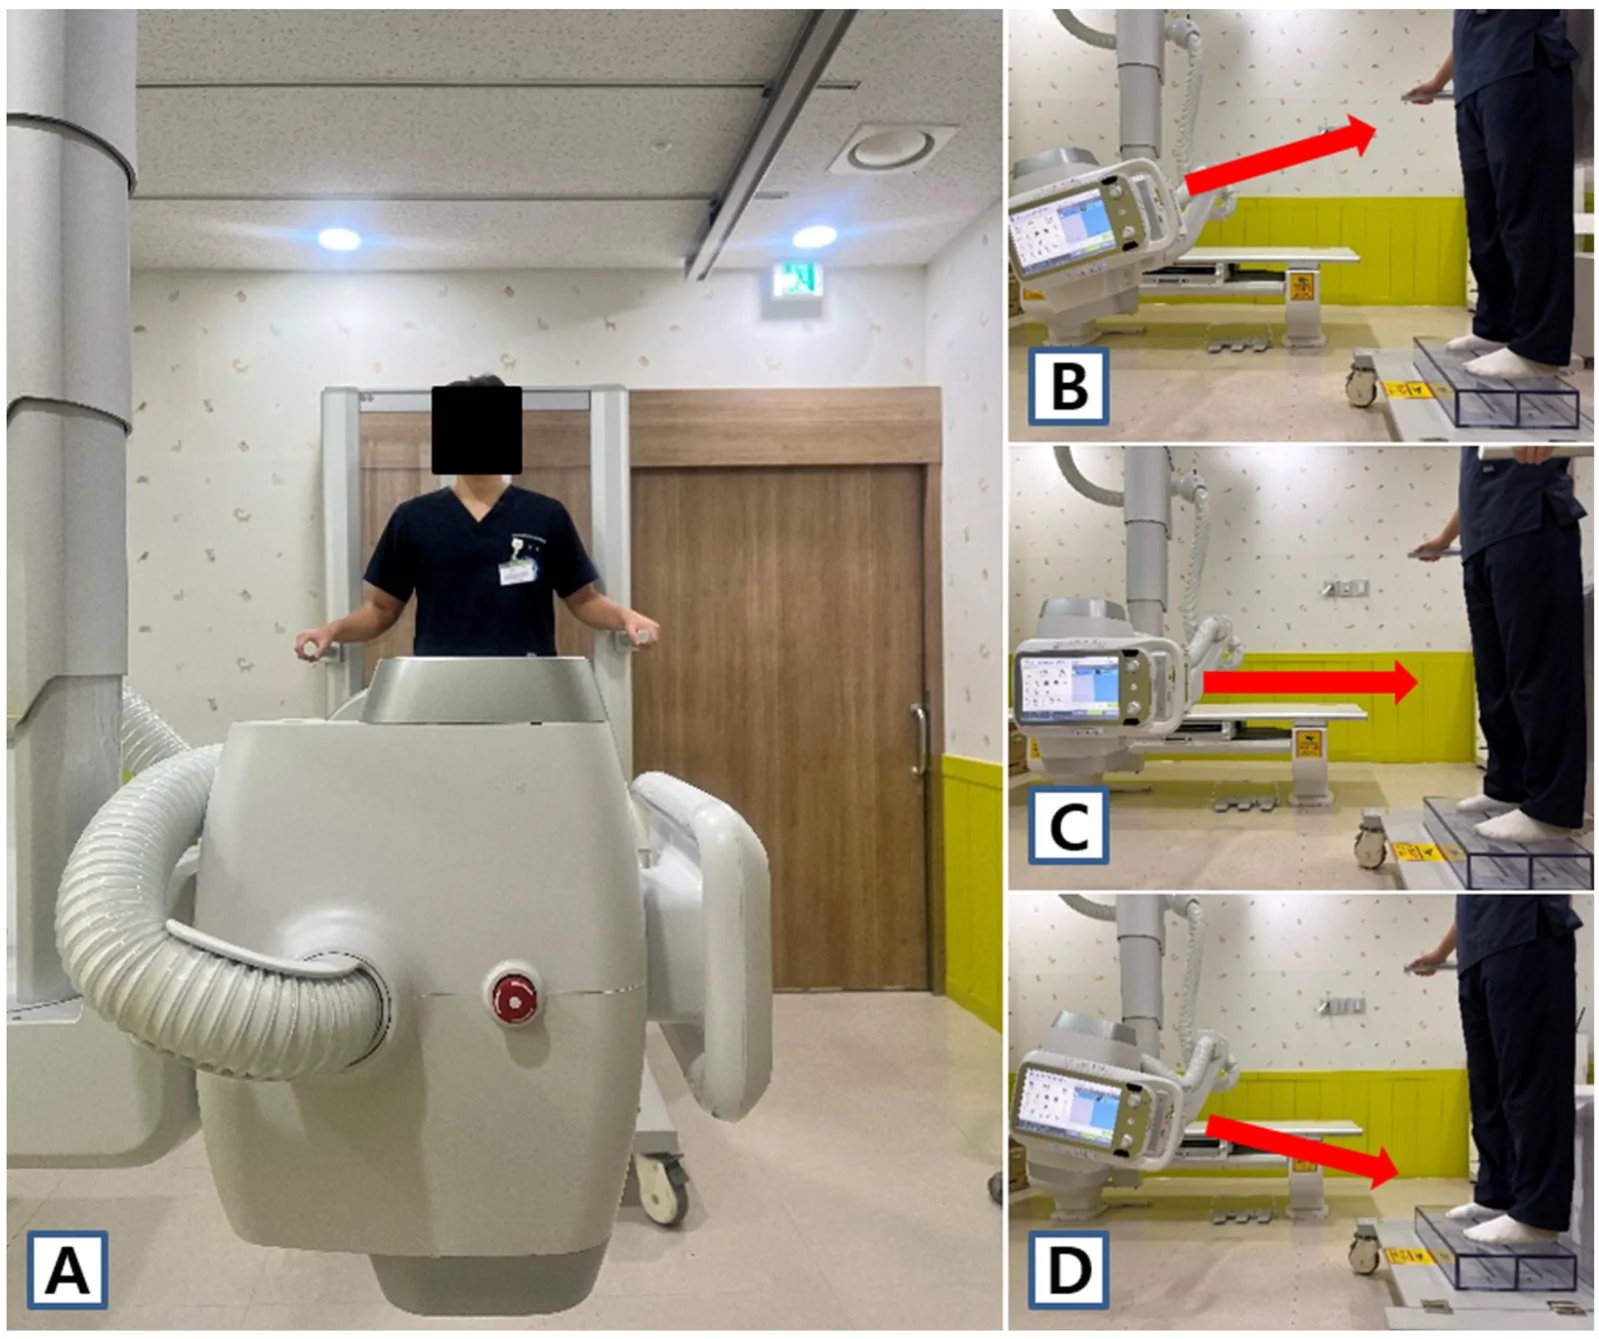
Lower extremity scanogram radiographic acquisition techniques. (A) shows the frontal position of the X-ray beam projector during scanogram acquisition. (B–D) show the lateral position and angulation of the beam projector during hip, knee, and ankle imaging, respectively. Red arrows in (B–D) indicate the direction of the X-ray beam.
